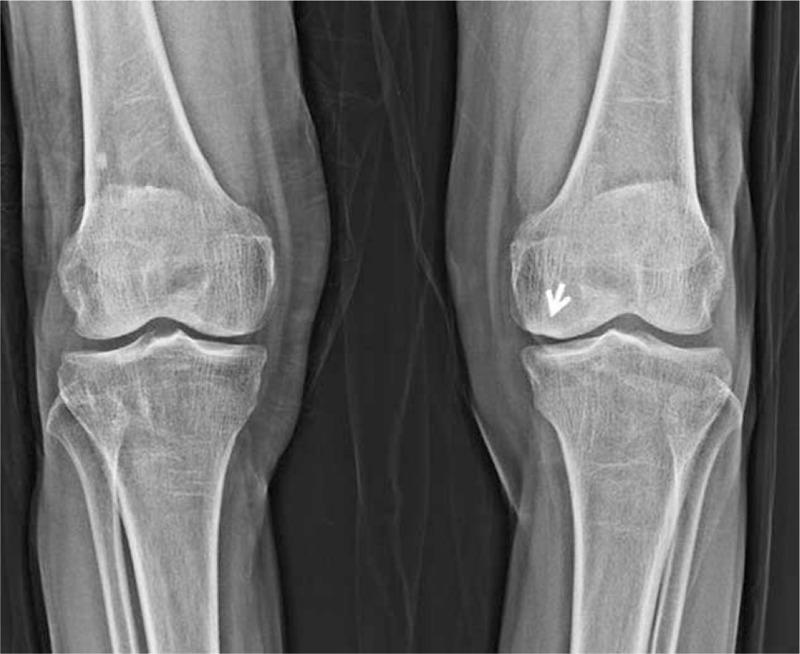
AP plain radiograph of the knee showed a scalloping subchondral lesion (arrow) of the medial femoral condyle with mild joint-space narrowing during intra-articular corticosteroid injection of the knee joint.
